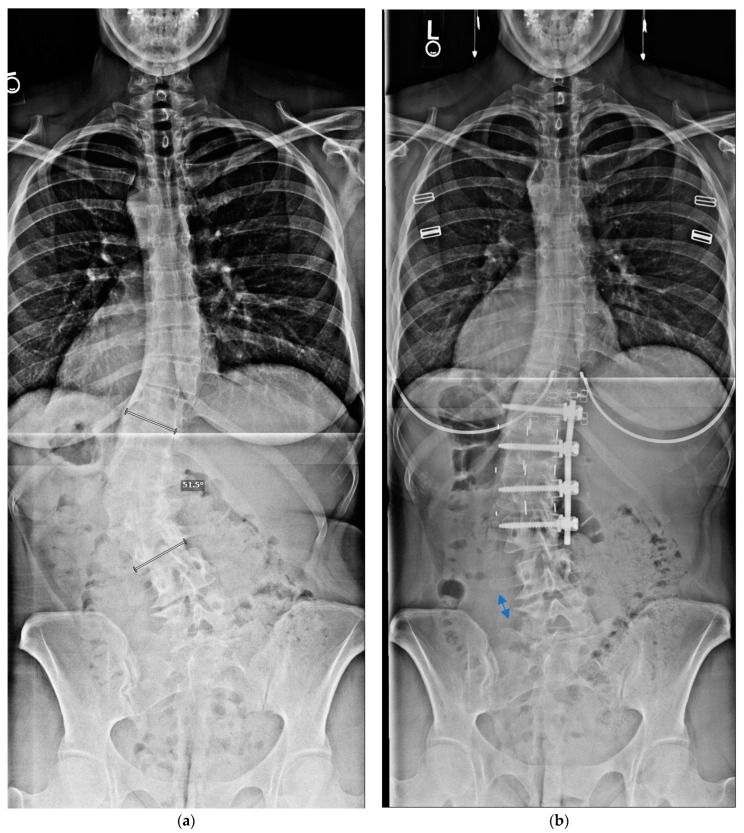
Preoperative and postoperative coronal radiographs (a,b) of a patient who had a resolution of left L5 pain symptoms after surgery. This demonstrates increased opening, indicated by the arrows, in the left L4–5 disc height in the fractional lumbosacral curve below the corrected T12–L3 fusion construct.
